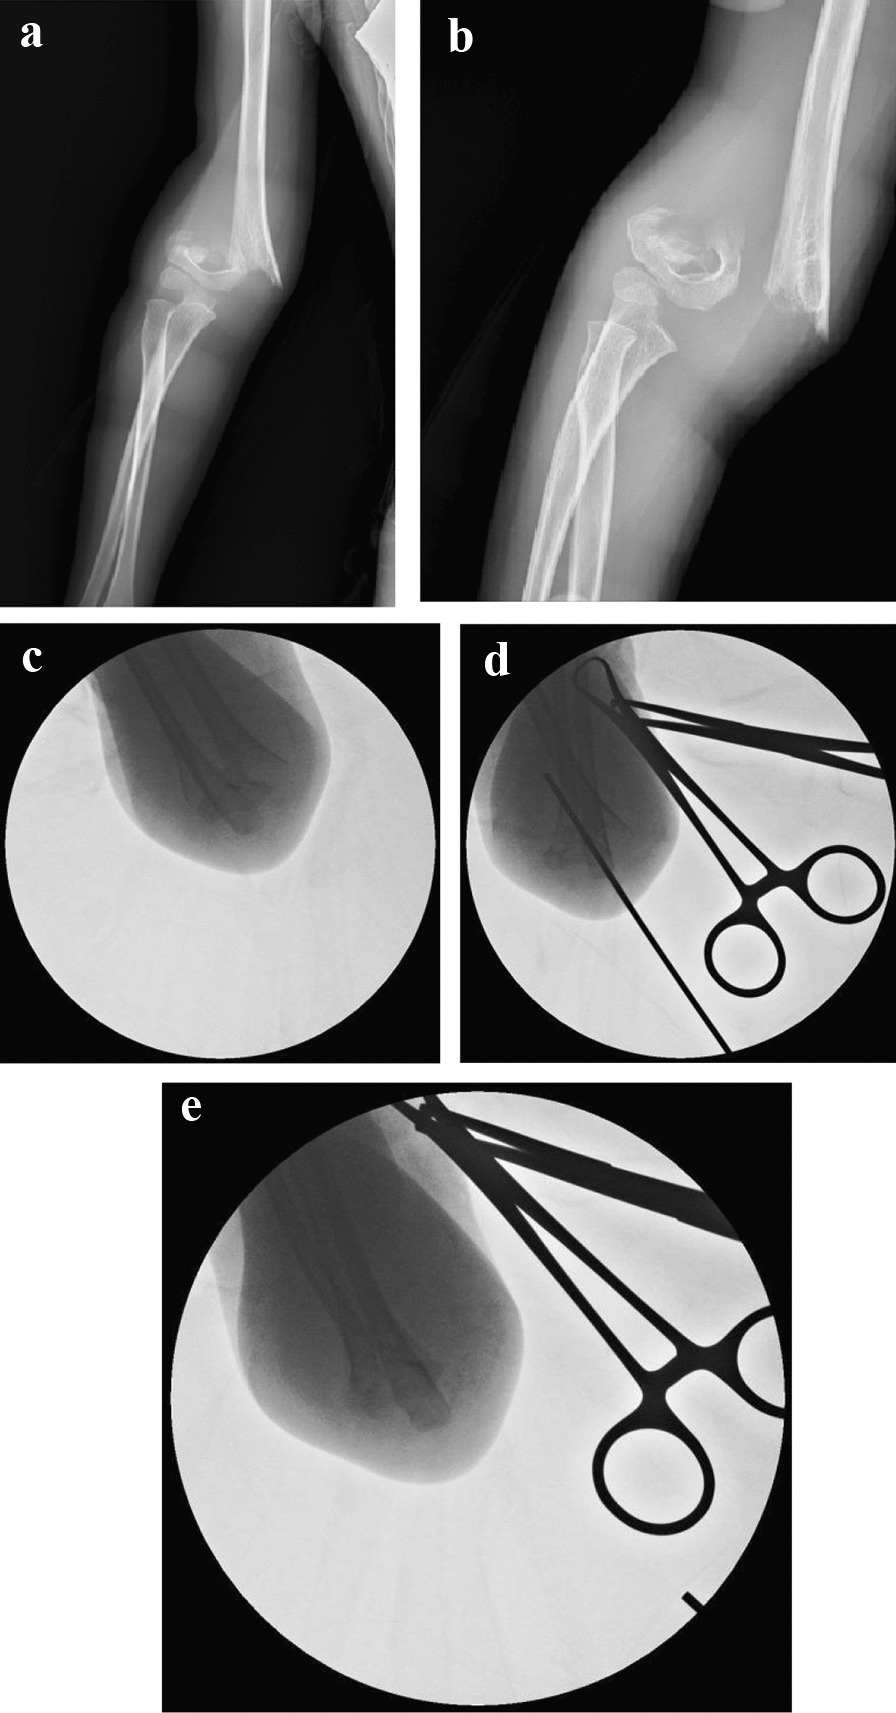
a–e The application of Kirschner Wire Prying Technique in practice (a type-IV SCFH in a 4.9-year-old boy). a, b Original X-ray of a posterolateral deviated SCFH. c After anesthesia, the surgeon could not make the position of fracture better by the gentle reduction. d, e The reduction gets better after using the Kirschner Wire Prying Technique on the AP view
